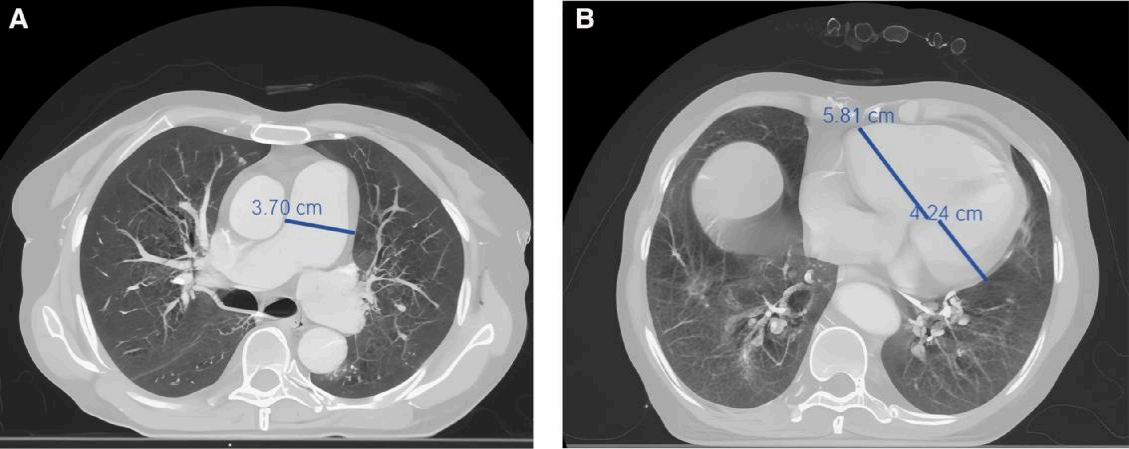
Chest CT scan showing a mobile hyperechoic mass in the right ventricle consistent with thrombus.
